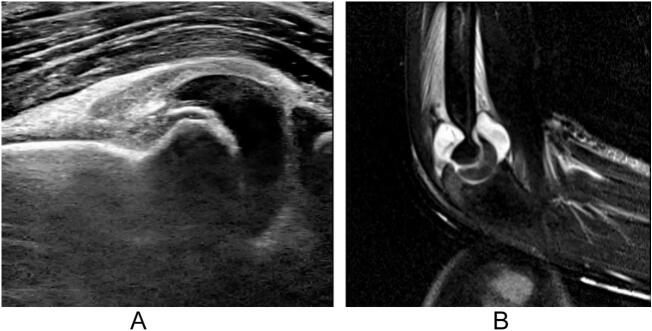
Ultrasound and magnetic resonance imaging (MRI) images show epiphyseal separation of the trochlea in the sagittal plane. (a) Ultrasound. (b) MRI.
